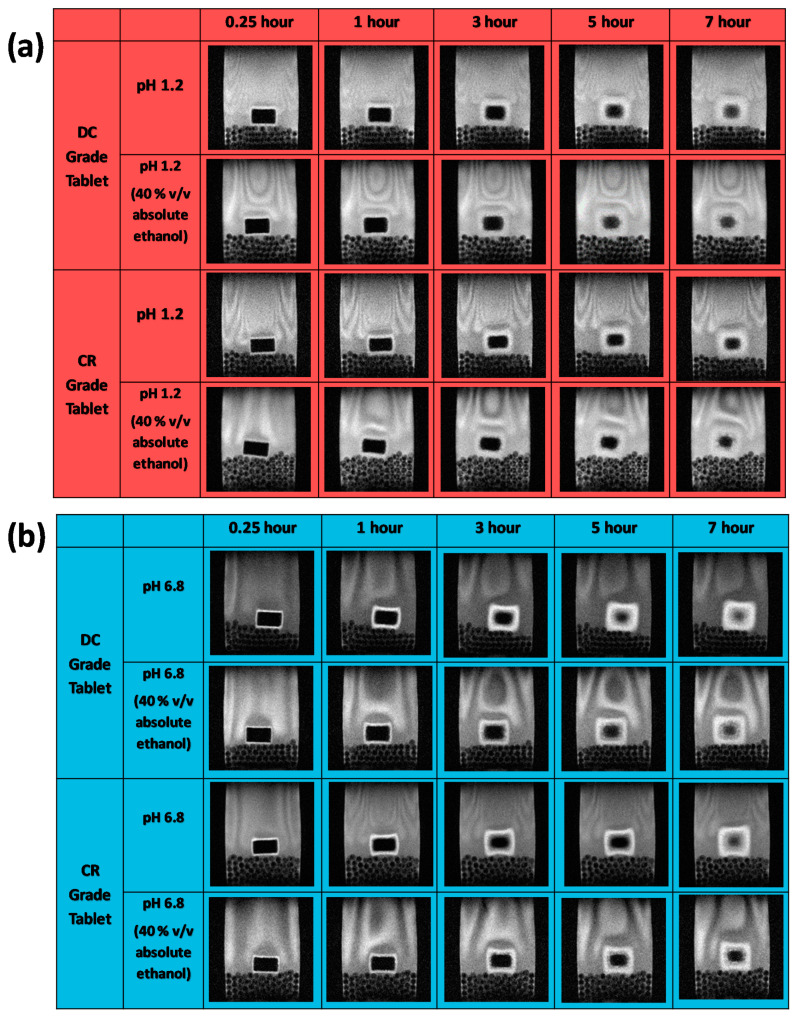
Magnetic resonance imaging of CR and DC formulations in (a) hydrochloric media with and without alcohol, (b) phosphate buffer media with and without alcohol.
